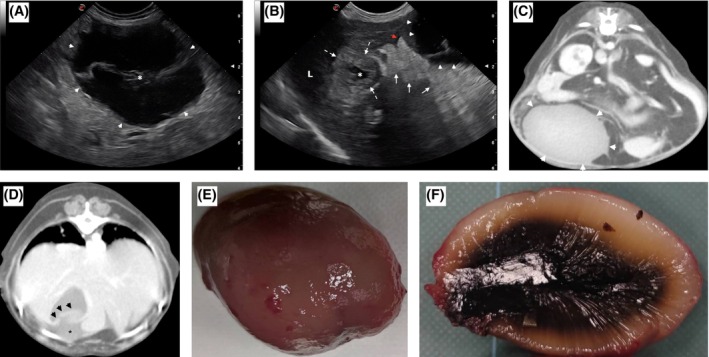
(A) Right sagittal, transabdominal ultrasonographic image of the migrating gallbladder mucocele (white arrowheads) diffusely hypoechoic with an irregular‐shaped hyperechoic centre (asterisk). (B) Right sagittal, transabdominal ultrasonographic image of the liver and gallbladder. Collapsed gallbladder lumen (asterisk), thickened gallbladder wall (dashed line), surrounding fat markedly hyperechoic (white arrows) and a very small amount of free fluid (red arrowhead) are evident. The mucocele can be partially seen (white arrowheads). L = liver. (C) Transverse computed tomographic scan of the gallbladder mucocele at the level of L1 (white arrowheads). (D) Transverse computed tomographic scan of the gallbladder at the level of T10. The gallbladder wall was thickened and irregular (black arrowheads). A tear in the gallbladder wall with content extravasation was evident (asterisk). (E) The migrating gallbladder mucocele after being removed surgically and (F) its section.
